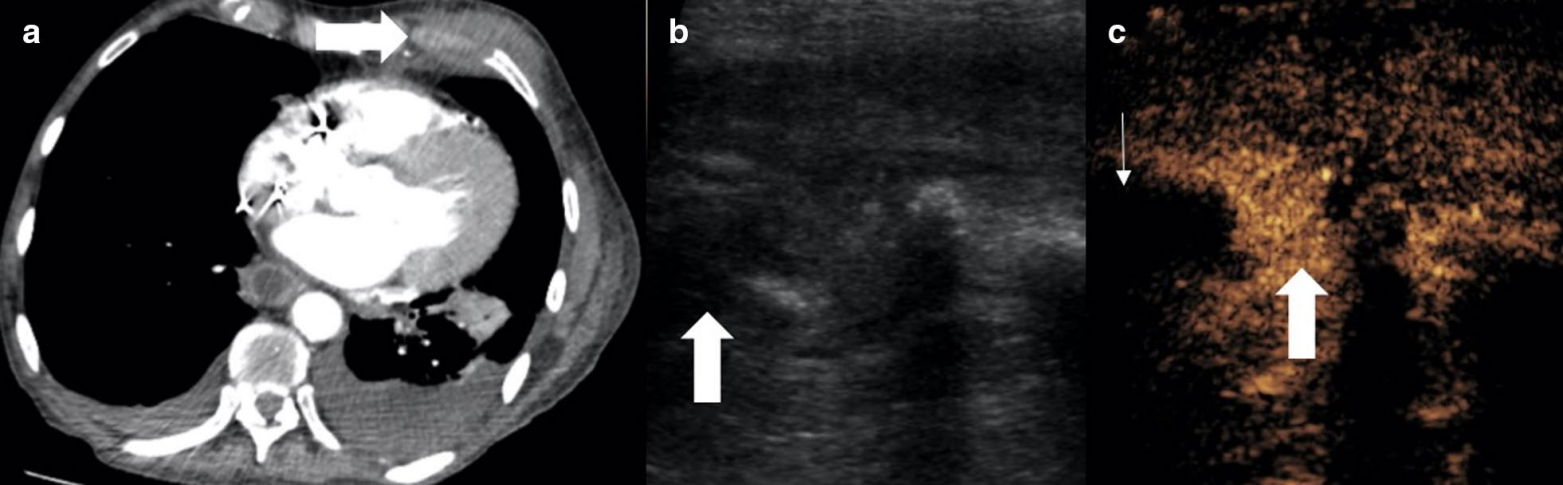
Patient with (a) CT examination showing an indeterminate rib lesion (arrow) and clinical sepsis. b, c Simultaneous B mode and CEUS better defined the lesion which was not well seen on B-mode ultrasound imaging (arrow) into a solid hyperenhancing component (thin arrow) and central avascular component (thick arrow). Fluid aspiration yielded pus of the fluid component and the biopsy was inflammatory indicating osteomyelitis
